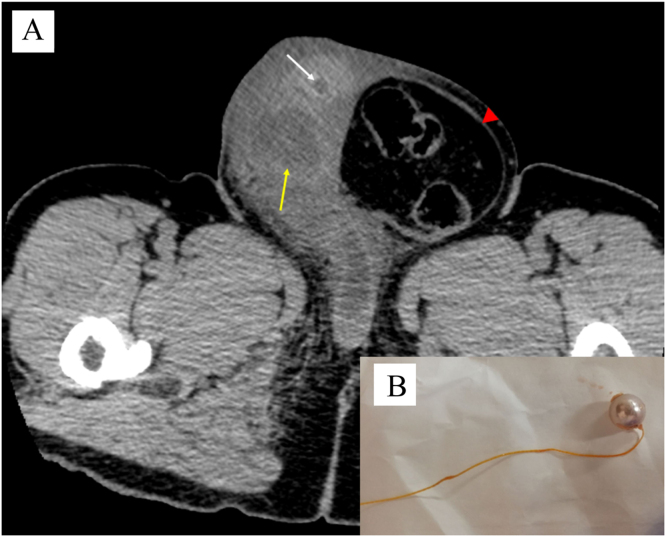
(A) Computed tomography showing a left inguinal hernia (red arrowhead), foreign body (white arrow) in the urethra, and irregular low-density area (yellow arrow), indicating an abscess in the penile corpus cavernosum. (B) The foreign body was removed and identified as a pearl with a thread.
